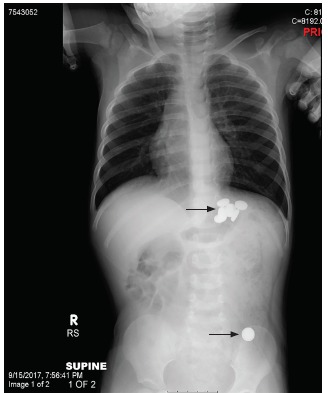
Supine radiograph showing multiple button batteries (arrows) approximately four hours after ingestion.
